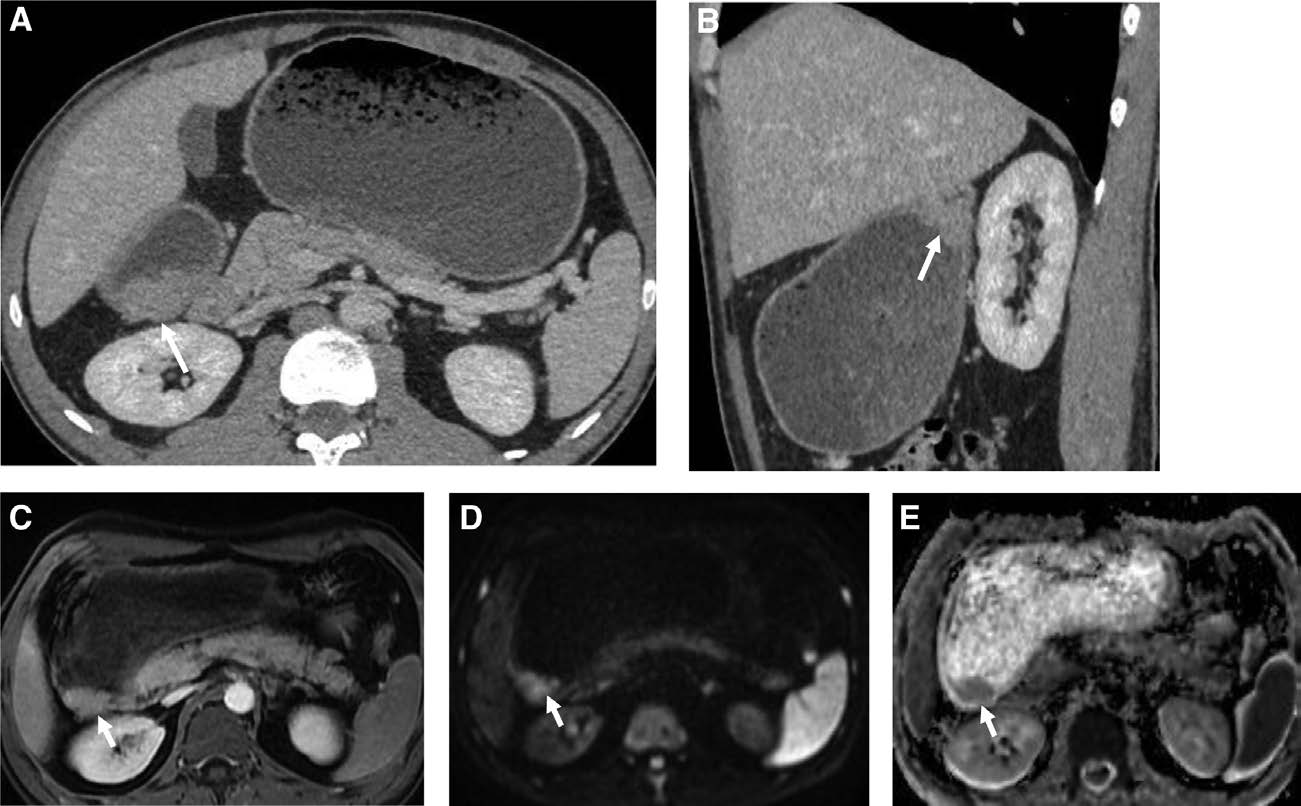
Imaging findings: (A and B) Abdominal CT scan axial and sagittal planes, portovenous phase, show gastrostasis caused by an infiltration of pylorus wall (arrow), (C–E) Abdominal MRI axial plane, there is a contrast enhancing (C) lesion (arrow) with a diffusion restriction (D–E) in the pylorus wall. MRI = magnetic resonance tomography.
